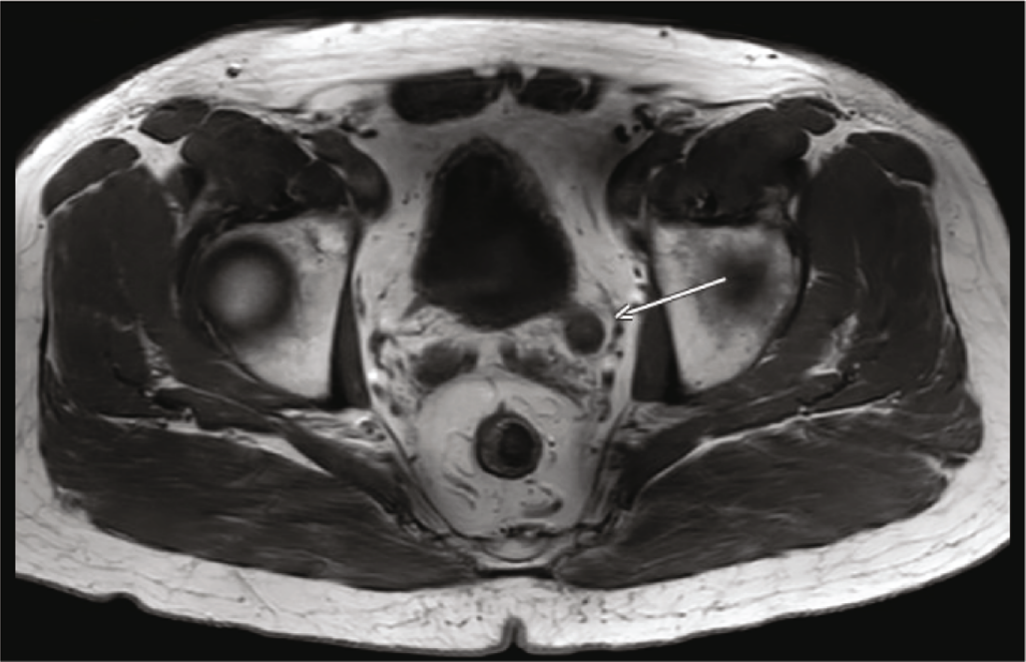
MRI with left distal ureteral recurrence (arrow).
